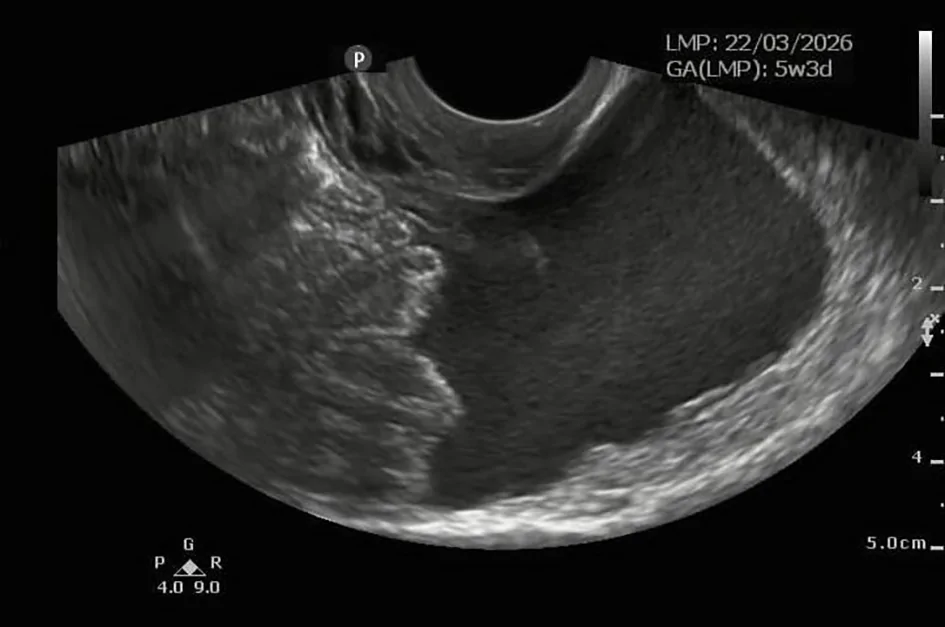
Free fluid within the pouch of Douglas identified on transvaginal ultrasound, suggestive of hemoperitoneum.
